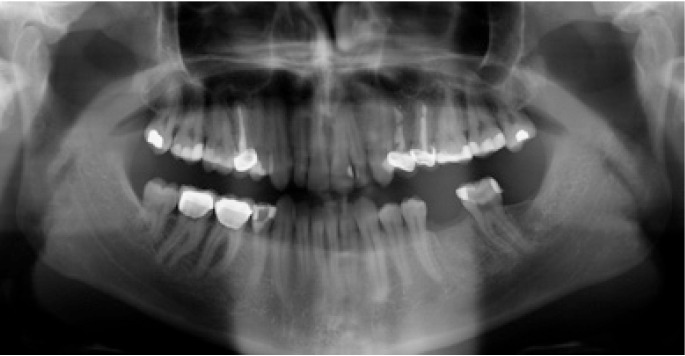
Preoperative panoramic X-ray view.
